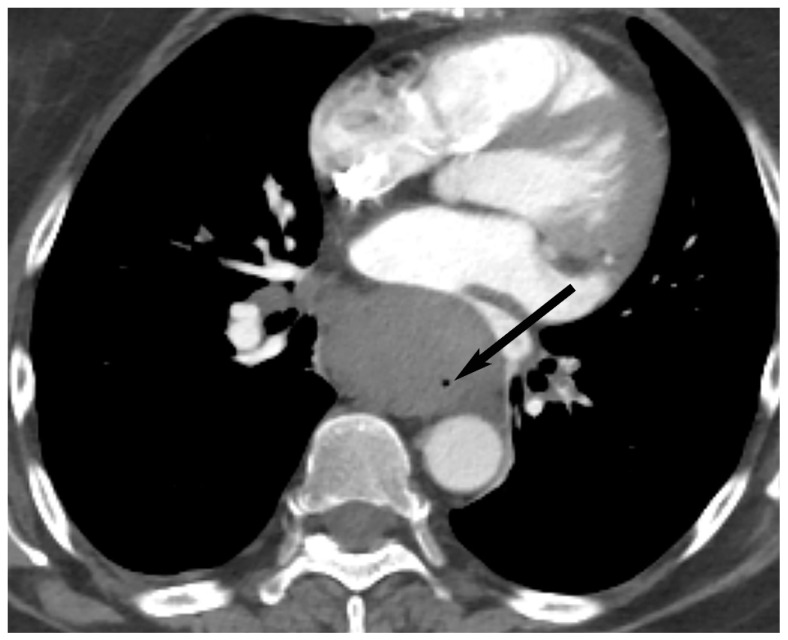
Esophageal carcinoma. CT shows a soft tissue mass in the visceral mediastinum inseparable from the esophagus (arrow). Biopsy showed esophageal carcinoma.
